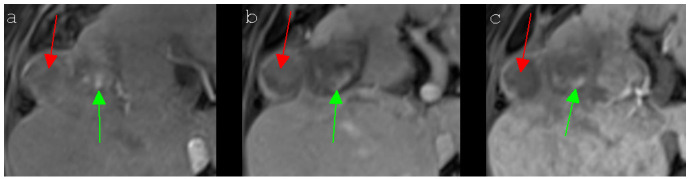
A 67-year-old man with a local tumor progression in the peripheral ablation zone (segment VIII/Iva) six months after IRE of an HCC, which presented atypical signal behavior in Gd-EOB-DTPA-based MR imaging. The nodular, exophytic HCC (red arrow) showed a slight peripheral enhancement and a central hypointensity in the contrast-enhanced T1 arterial phase MR image (a), the typically known hypervascularization was lacking, however. The signal intensities of the HCC remained unchanged during the contrast-enhanced T1 portal venous phase (b) and the contrast-enhanced T1 delayed phase MR image (c). A typical “wash-out” could not be observed. The central part of the ablation area showed several hyperintense spots (green arrow), which could be detected in all presented MRI sequences (a–c), attributable to post-interventional hemorrhagic transformation.
